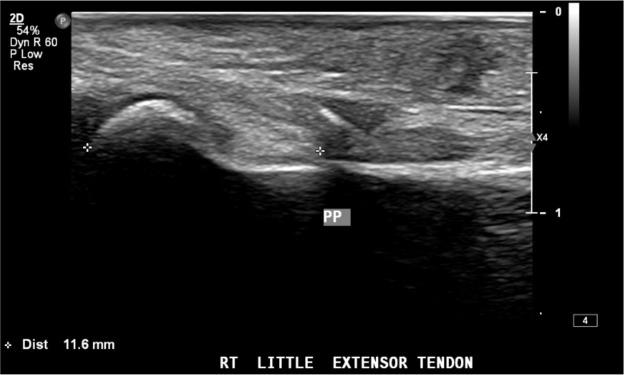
Ultrasound of flexor tendons (longitudinal, Case 1).
